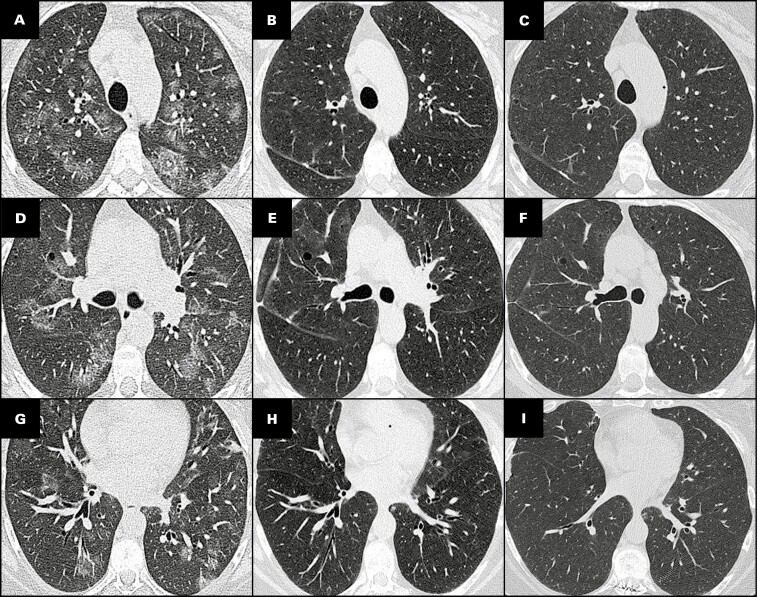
A-I, Imaging follow-up in smoking-related interstitial fibrosis. All images are from case 5 (48-year-old woman). A, D, G, Upper, middle and lower lobes from initial computed tomography (CT), showing nodular bronchocentric ground-glass opacities. B, E, H, Upper, middle, and lower lobes from CT at approximately 4 years of follow-up, showing resolution of ground-glass opacities. C, F, I, CT at nearly 8 years (95 months) of follow-up, showing stable findings.
